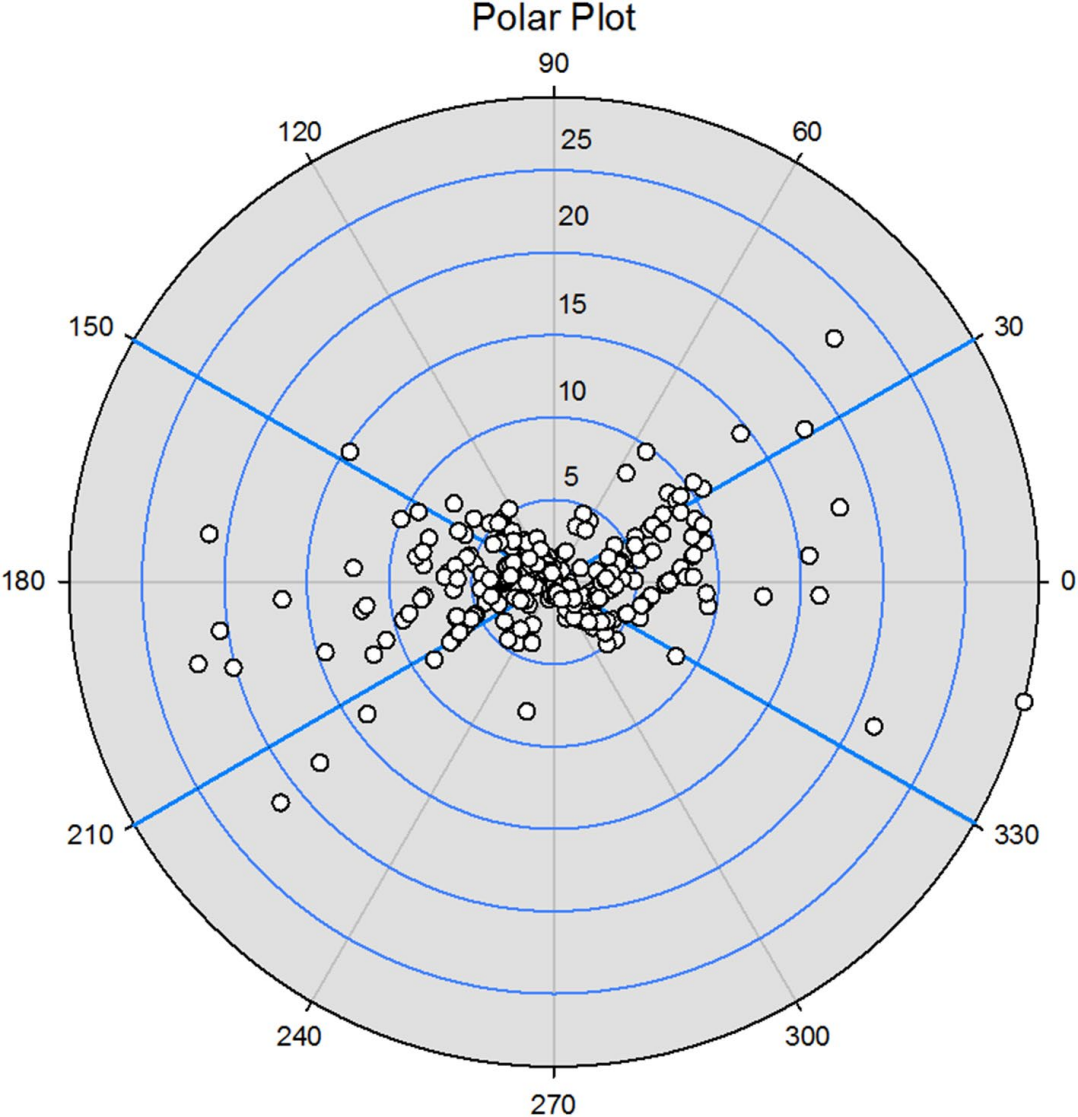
Polar plot analysis on trending ability of changes of Hb measured by transesophageal dPPG signal against the blood gas analysis
